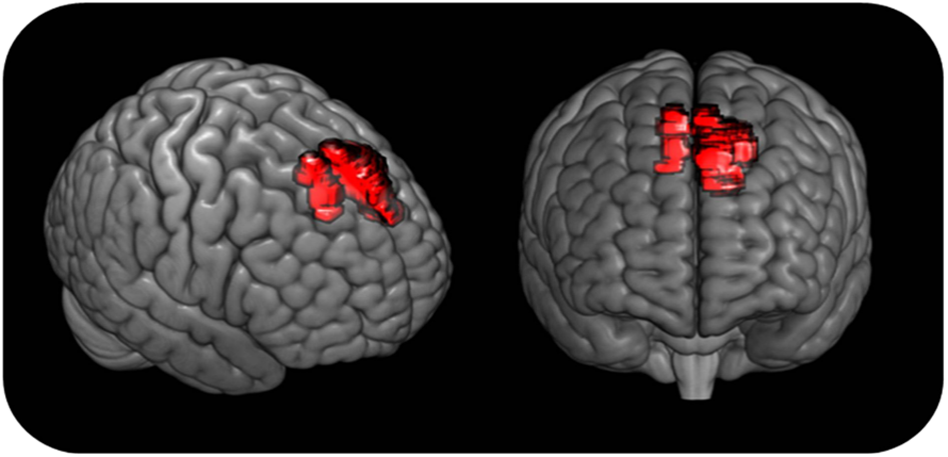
3D reconstruction of the defendant’s exact brain lesion. Based on the CT scan, the brain lesion was manually traced by one of the authors (CS) on the MRIcro template. The traced lesion was then overlaid to the 3D render provided by MRIcroGL software (http://www.cabiatl.com/mricrogl/)
